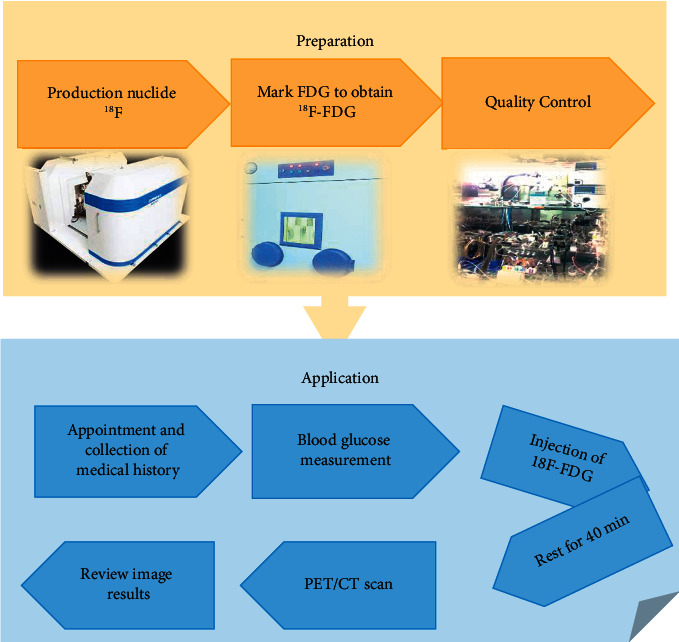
Preparation and application process of the ^18^F-FDG drug.
